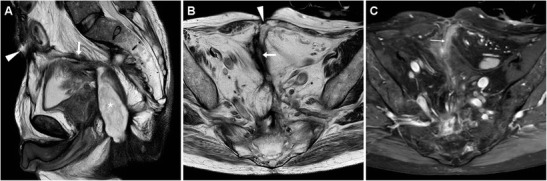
MRI diagnosis of post-surgical ECF in a 65-year-old male with long-standing fistulising CD, previous abdomino-perineal resection for perianal fistulas complicated by anal squamocellular carcinoma (ASC). On T2-weighted images, the fluid-filled ECF (arrows) was seen coursing ventrally to reach the ventral EFO (arrowheads) at the site of past surgical incision, from the apex of a large T2-hyperintense mass (* in A) corresponding to recurrent ASC. Post-gadolinium fat-suppressed T1-weighted image (C) showed mucosal hyperenhancement (thin arrow) along the fistula track
